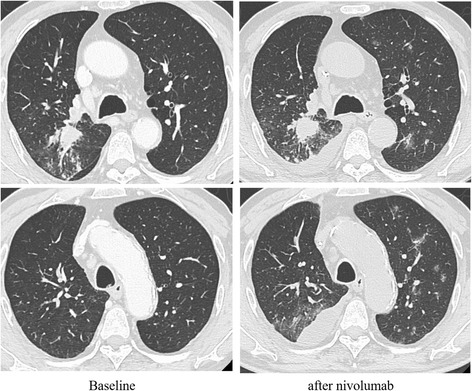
Clinical responses to nivolumab. Chest CT scan showed progressive disease after nivolumab
